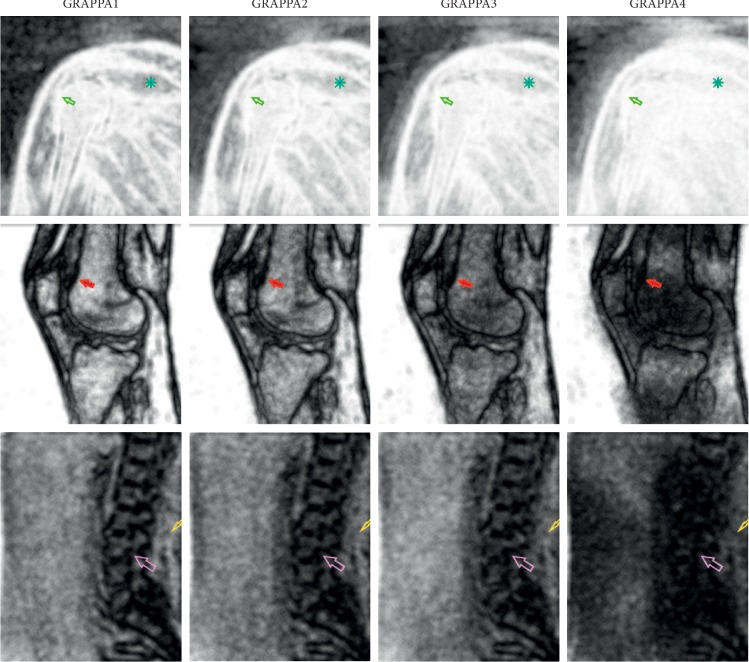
Texture feature maps (GLCM) of the MR images of the knee made with gradually reduced acquisition times (GRAPPA1–4). Image representation is shown on consecutive columns (left to right). The images show a gradual fading of the anatomical object features with a decreasing acquisition time. Note the degradation of the bone outline, which is a structure that is the most persistent on the image as indicated by arrows. The muscle tissue is indicated with an asterisk (upper row) and a small arrow (bottom row). The following texture features are present: GLCM entropy, histogram max, and GLCM-inverse difference moment.
